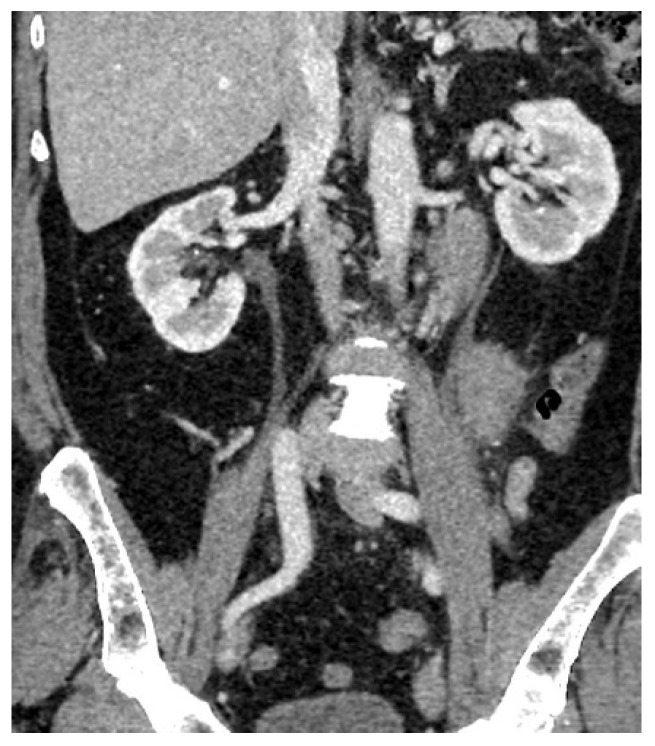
Abdomino-pelvic CT with contrast substance, coronal plane sections: Adenopathies along the path of the left testicular vein.
